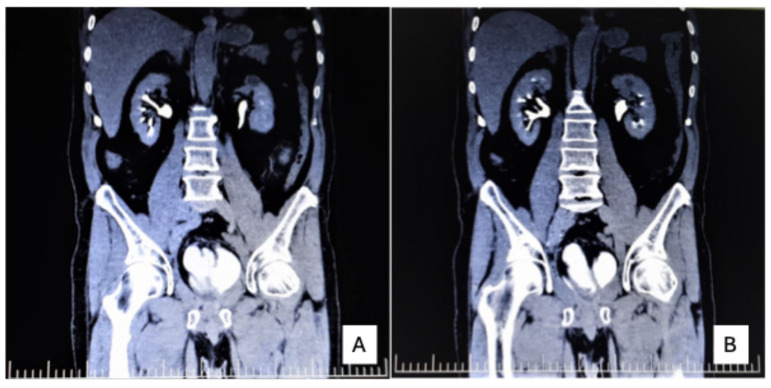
Contrast-enhanced Uro-CT demonstrating postoperative findings and inflammatory complications. (A) Coronal contrast-enhanced CT reconstruction showing focal hypodense areas in the left kidney, consistent with acute pyelonephritis, along with a cortical renal cyst. (B) Coronal reconstruction demonstrating the orthotopic ileal neobladder within the pelvic cavity, with normal postoperative appearance.
